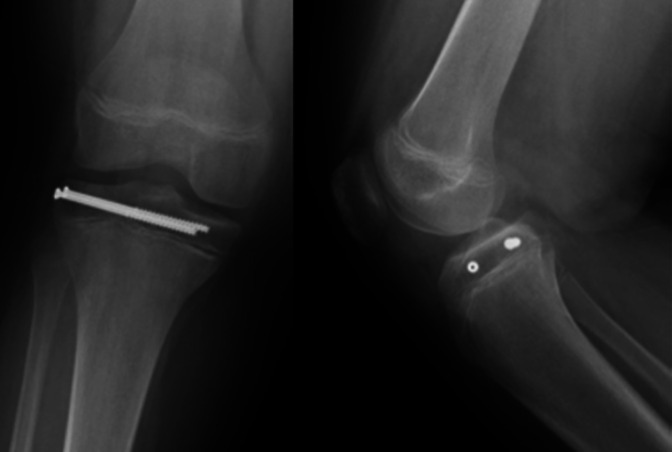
Six-week radiographs show healing of the fractures in the anatomic position.
